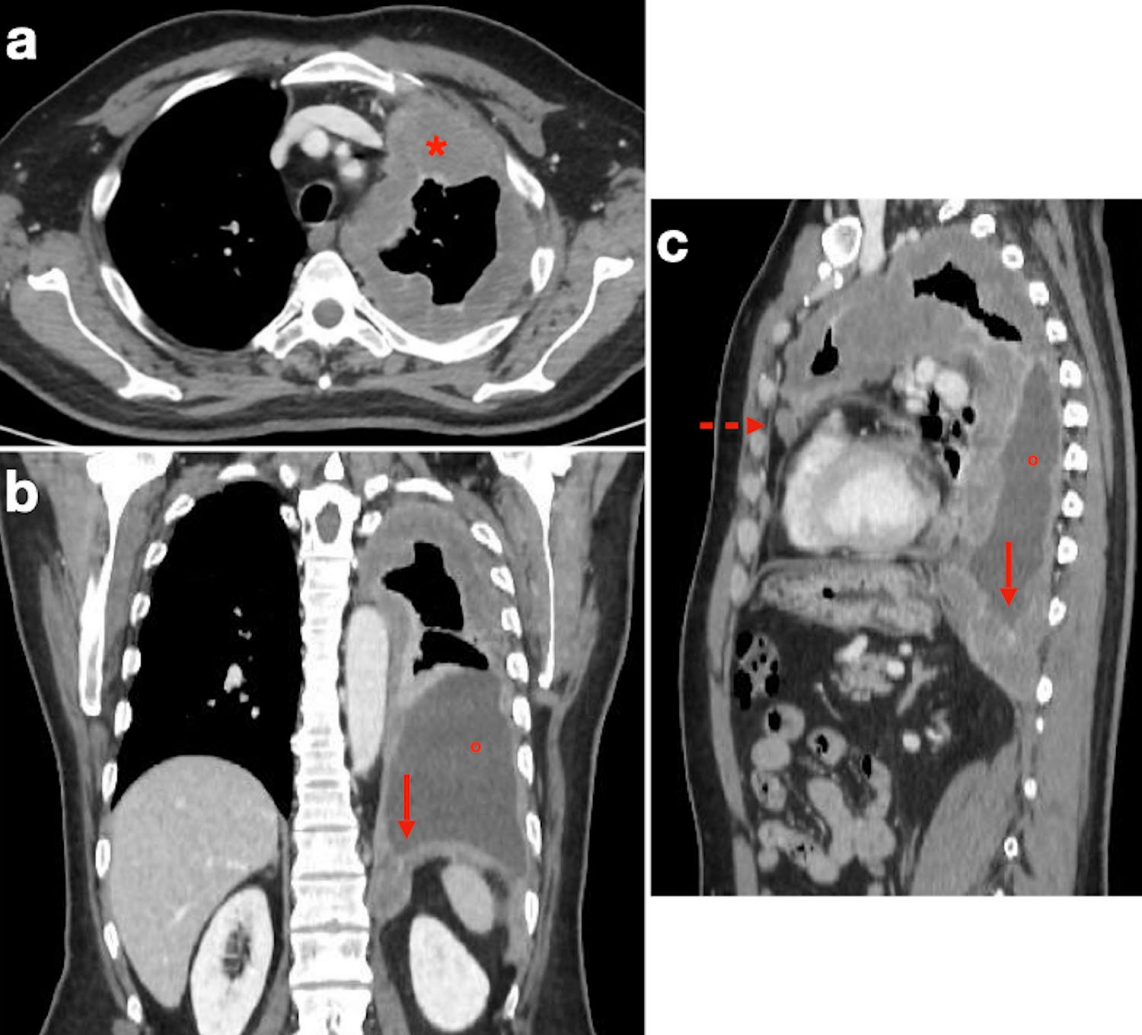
72-year-old man with history of asbestos exposure undergoing multidetector CT examination for PM staging. a Axial image shows diffuse, rind-like soft tissue with contrast enhancement that determines marked pleural thickening and left lung encasement (asterisk). b Loculated pleural effusion (circle) is present, along with infiltration of the ipsilateral hemidiaphragm (arrow). c Infiltration of the epicardial fat (dashed arrow) with no clear demarcation from the pericardial layer can also be seen
